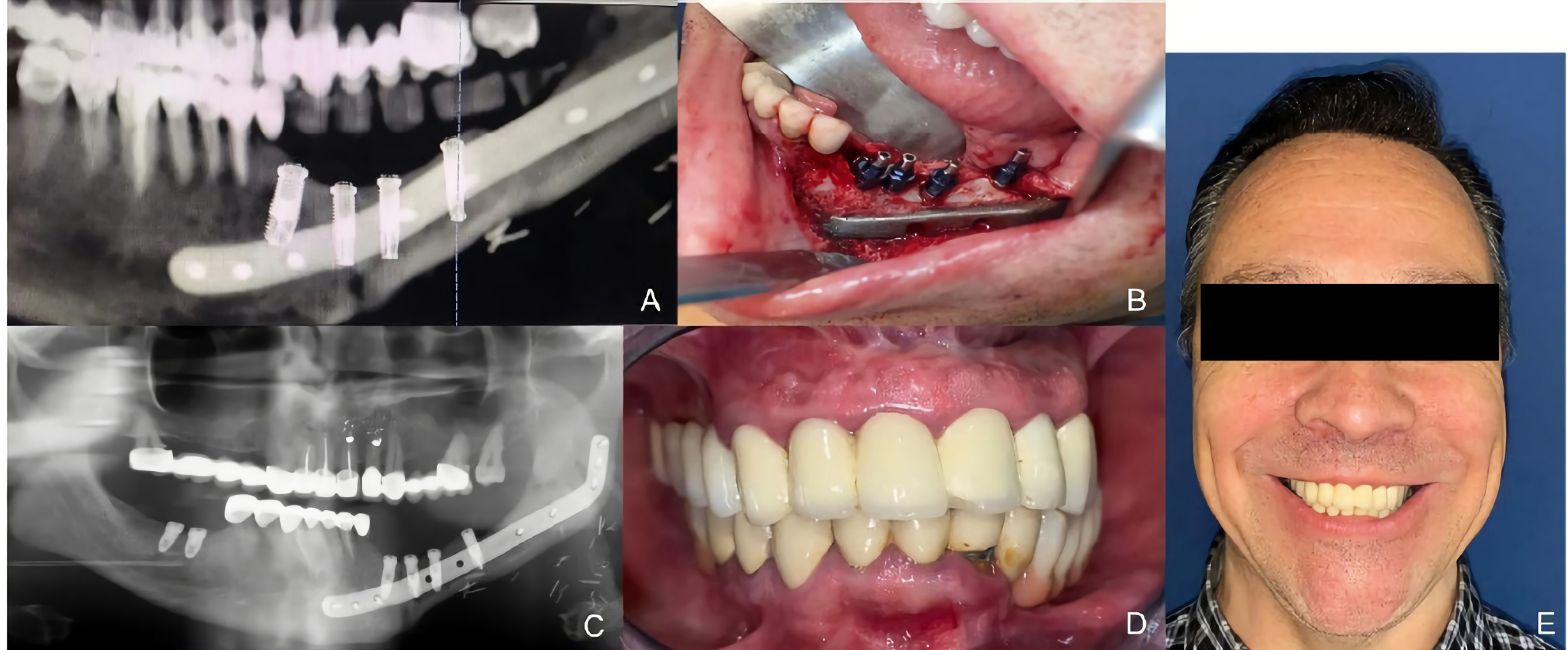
(A) Virtual planning of dental implants. (B) Intraoperative implant placement through dynamic navigation. (C) Panoramic radiograph after surgery showing a high accuracy compared with the virtual planning. (D) Prosthetic rehabilitation with implant fixed prosthesis. (E) Aesthetic and functional result.
